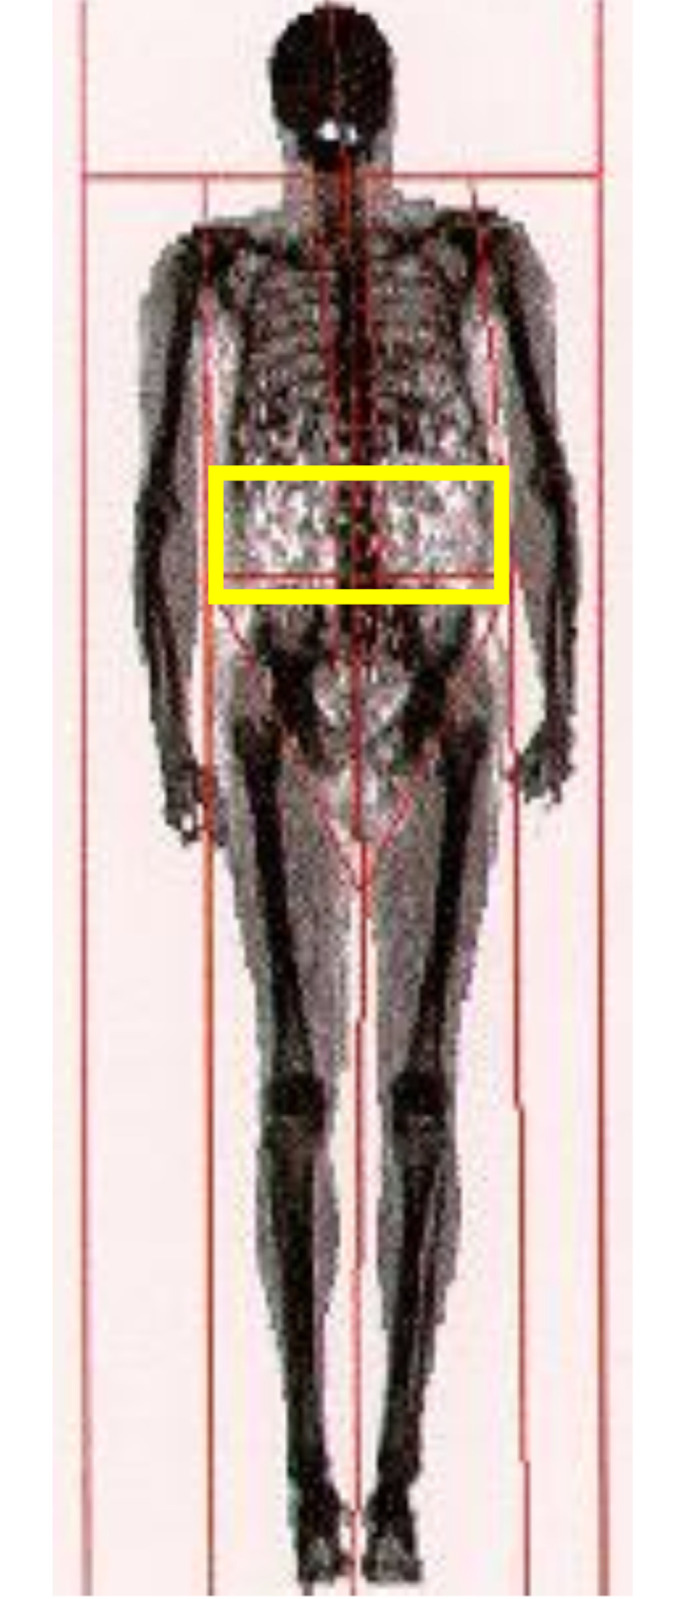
Measurement of body composition via dual x-ray absorptiometry (DEXA). A rectangle was drawn on the digital image bounded superiorly by a horizontal line identifying the T12/L1 intervertebral space, inferiorly by a horizontal line denoting the L4/L5 intervertebral space, and bilaterally by connecting the two horizontal lines in a region free of tissue. Abdominal adiposity, DXA L1-L4, was defined as the fat mass within this region.
